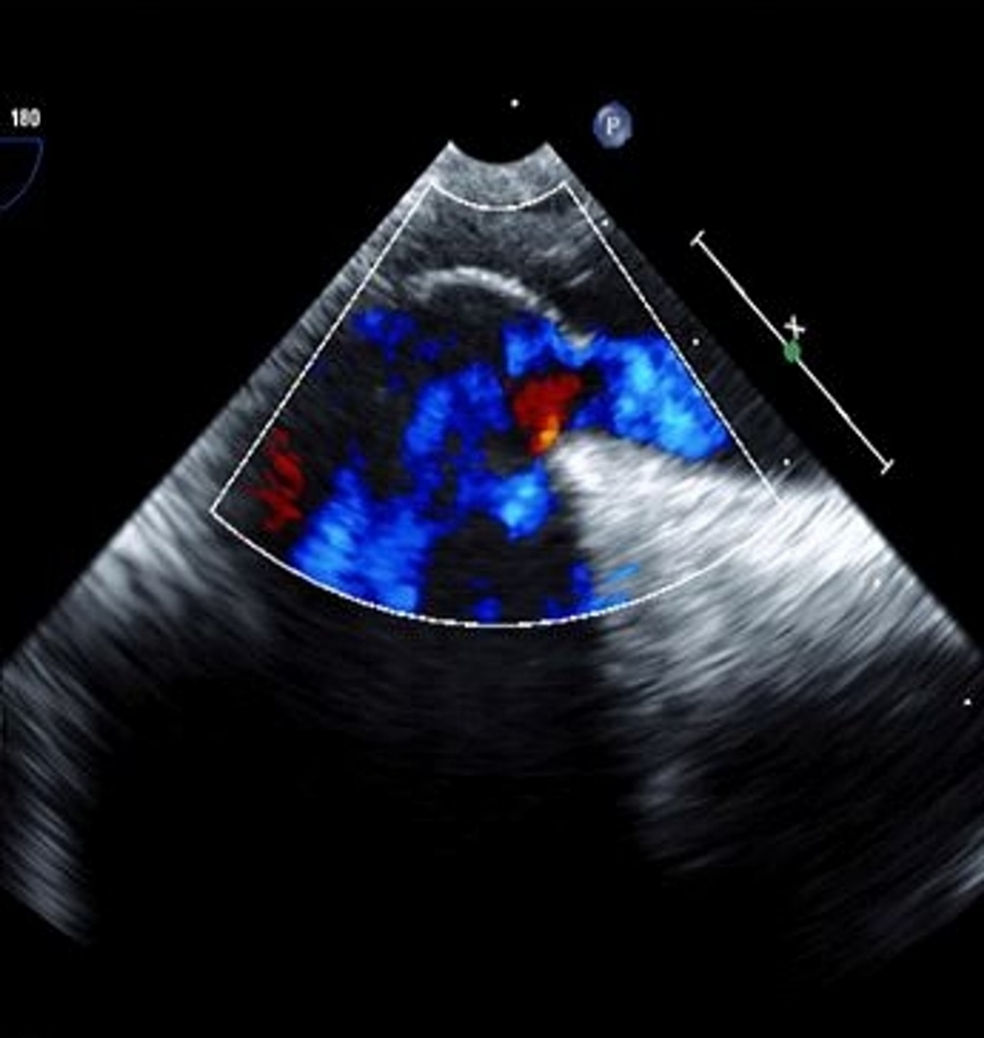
Transesophageal bicaval view (at 90°) showing the tunneled patent foramen ovale with color Doppler demonstrating right to left shunt.
